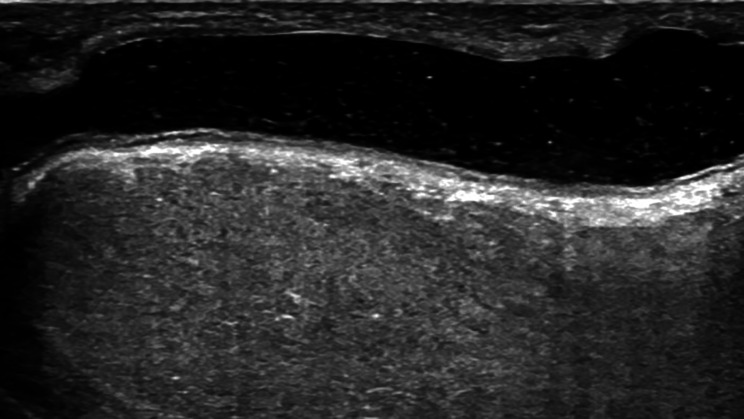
An ultrasound image of a patient with no symptoms after hypospadias surgery
